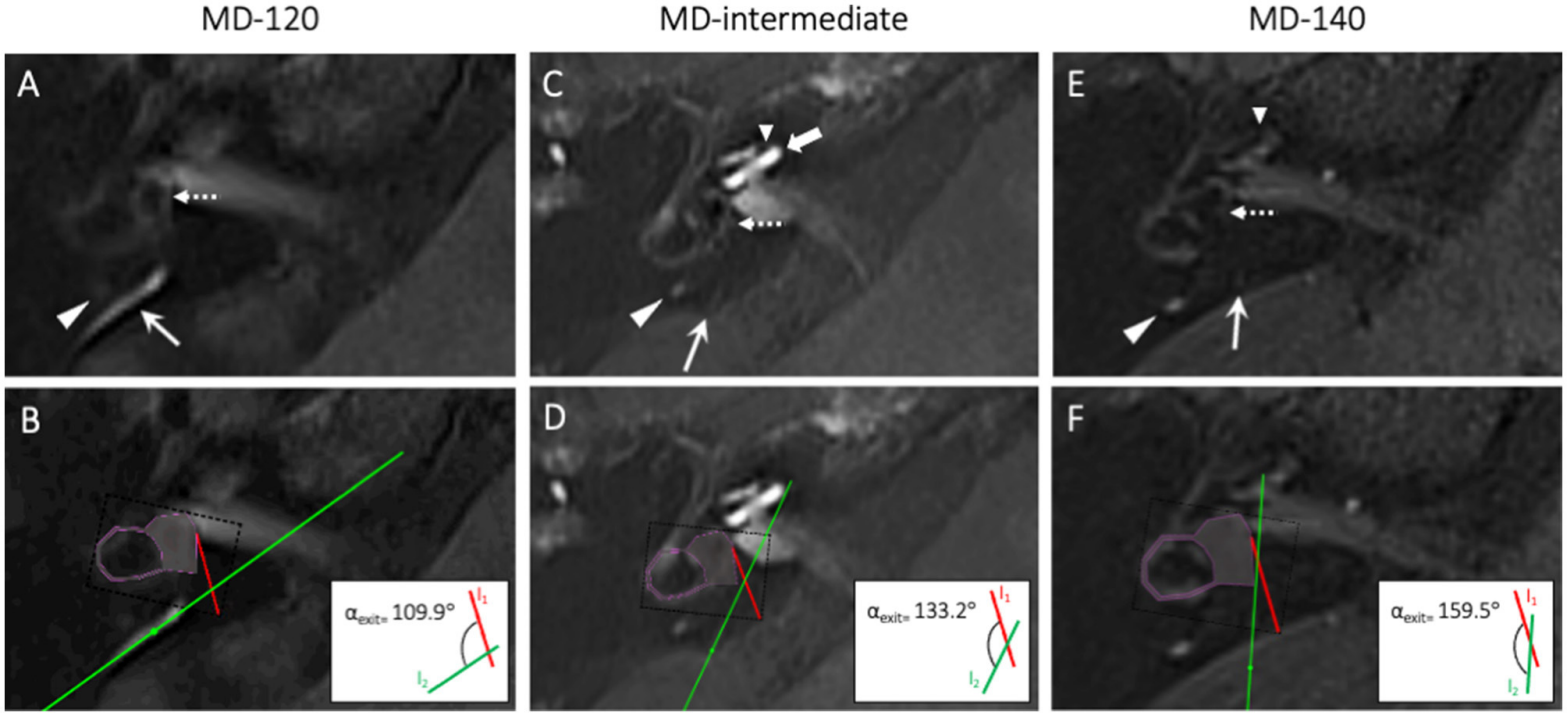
Angular trajectory of the ED-ES system in definite MD cases with MD-120 (A, B), MD-intermediate (C, D), and MD-140 (E, F) morphologies. (A, C, E) axial 4 h-delayed Gd-enhanced 3D FLAIR MRI, the long arrows indicate the ED-ES system in the opercular region. The ED-ES system lies in close proximity to the posterior semicircular canal (long arrow head). (B, D, F) show the corresponding ATVA by fitting a predefined shape (magenta shape) into the bony boundaries of the vestibule and horizontal semicircular canal. The red line (I_1_) is attached to this shape at a fixed angle of 14°, representing the entrance angle (α_entrance_) of the proximal ED in the temporal bone at its origin from the vestibule. The green line (I_2_) was fitted parallel to the trajectory along which the ES exits the temporal bone. The exit angle (α_exit_) of the ED-ES system was calculated by the software as the angle between I_1_ and I_2_. Note the presence of vestibular hydrops in all cases (dashed arrow in A, C, E), cochlear hydrops in the MD-intermediate and MD-140 cases (short arrowhead in C, E), as well as increased perilymphatic enhancement in the MD-intermediate case (thick arrow in C).
